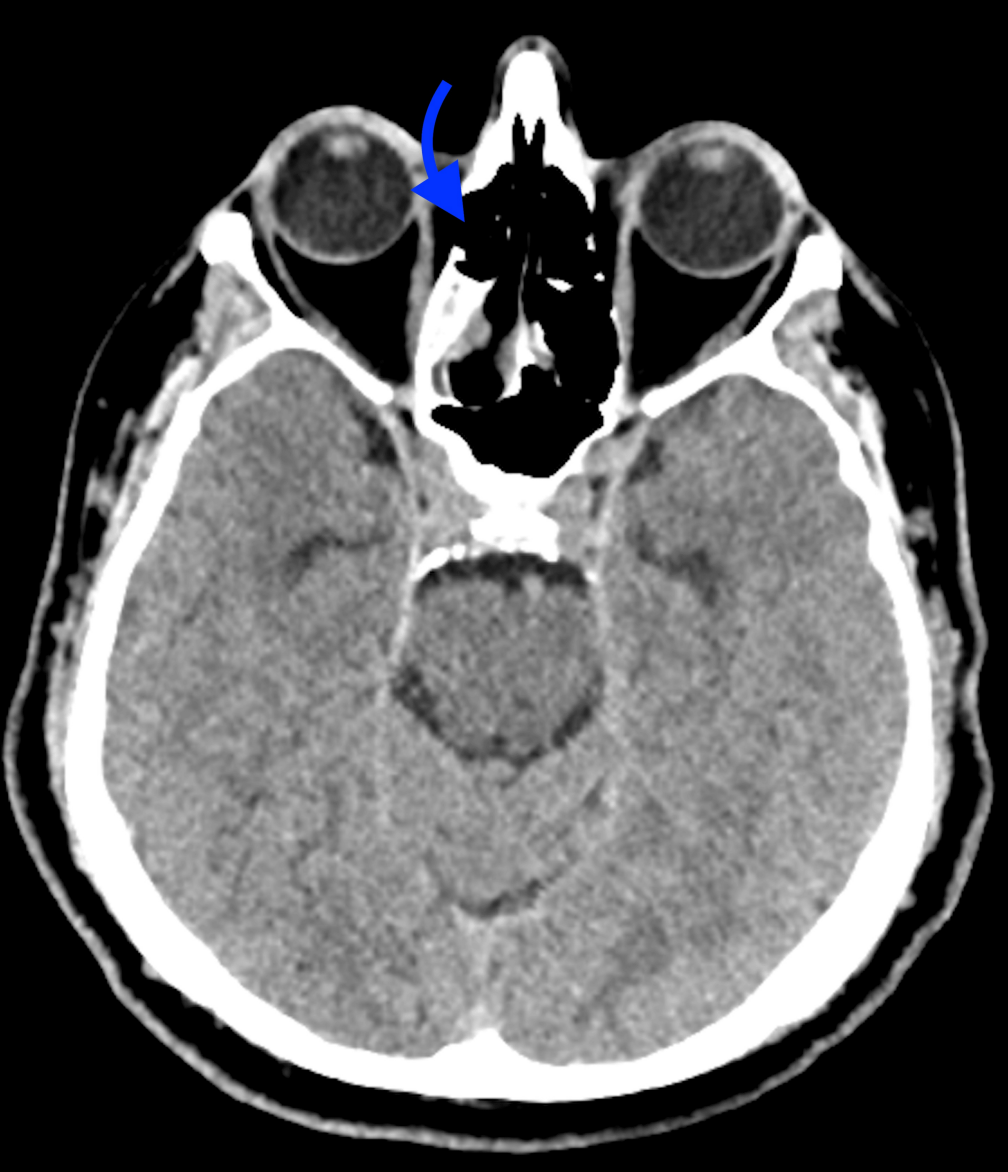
Axial CT head without contrast on postoperative day 2 - image 2 The image demonstrates a right cribriform plate defect after endoscopic sinonasal tumor resection (arrow) CT: computed tomography
